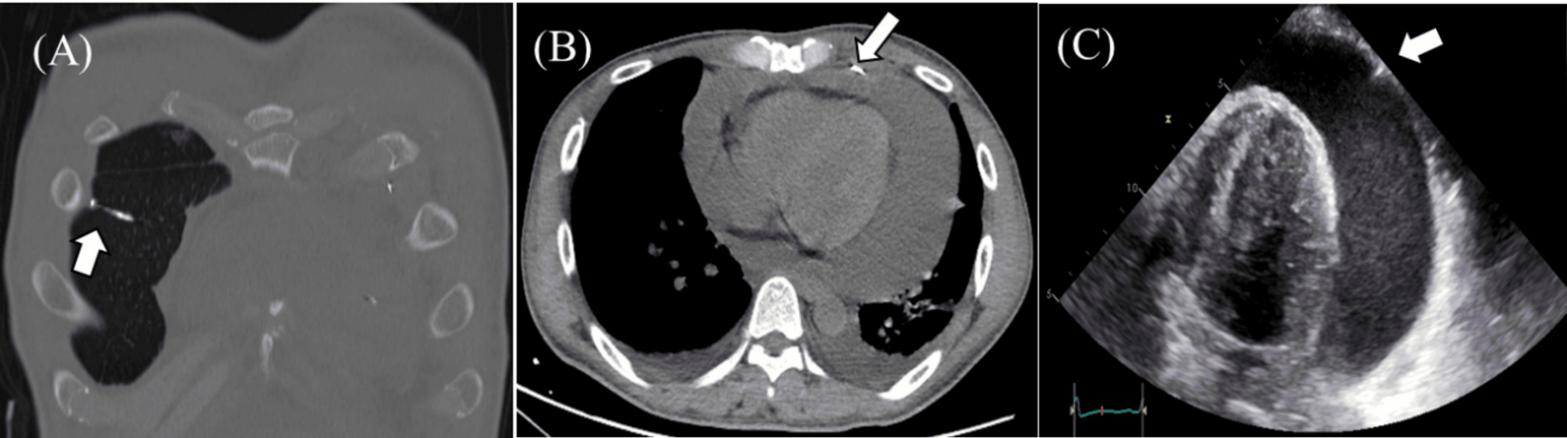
Chest computed tomography (CT) and cardiac ultrasound images at admission. (A) CT revealed a needle (white arrow) in the right lung parenchyma.
